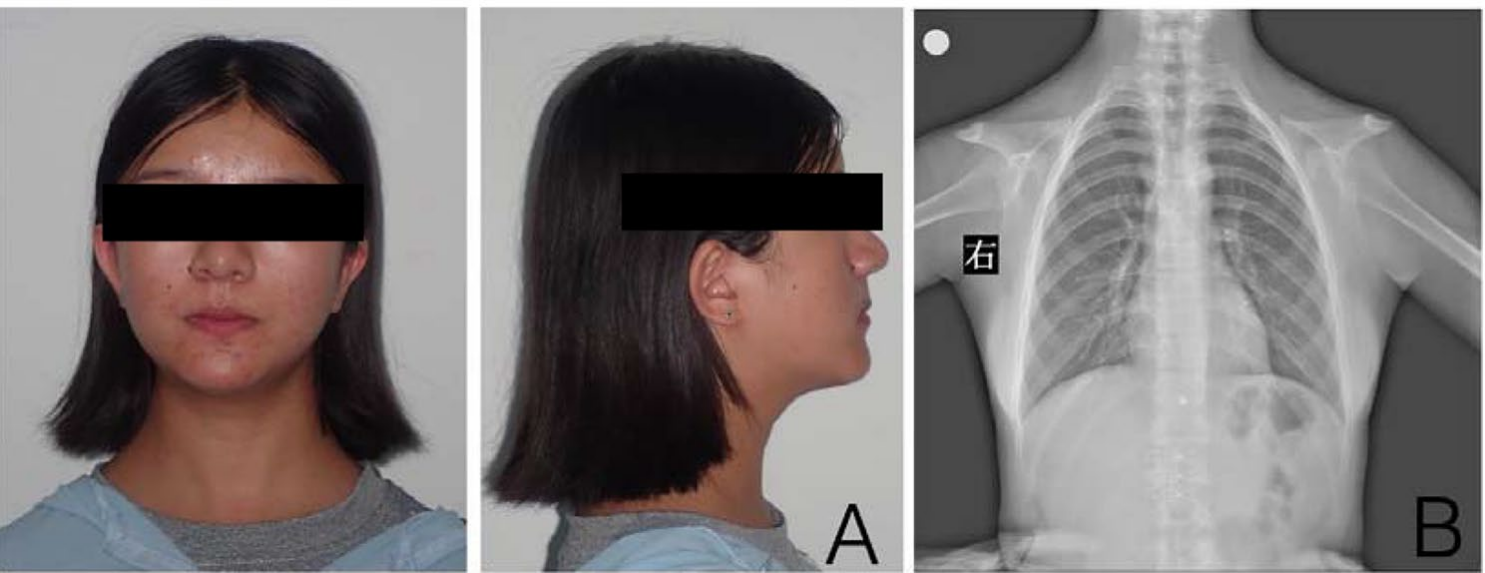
(A) Extraoral photographs showing the features of CCD patient: The skul is brachy-cephalic, with pronounced frontal and parietal bossings and the maxilla and zygomas are under developed. The bridge of the nose is broad and depressed, with ocular hyperterlorism; (B) Radiographic features of CCD subjects. Note the absence of clavicles shown by approximation of shoulders
